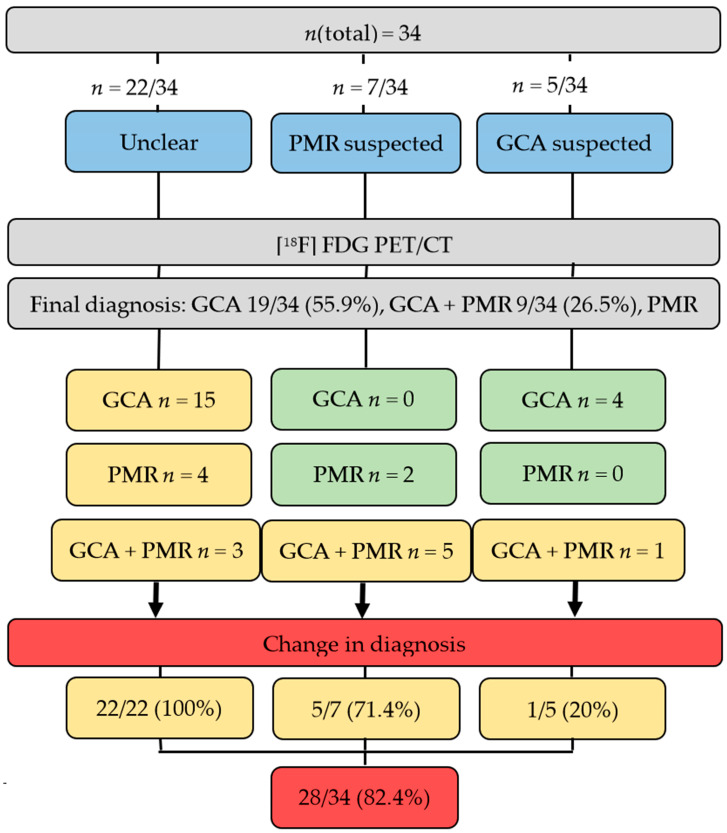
Thirty-four patients were examined by [^18^F]fluorodeoxyglucose (FDG) positron emission tomography/computed tomography (PET/CT) with unclear diagnosis or suspected giant cell arteritis (GCA) or polymyalgia rheumatica (PMR). In 28/34 (82.4%), [^18^F]FDG PET/CT was able to establish the diagnosis (red). In the remaining 6/34 (17.6%), [^18^F]FDG PET/CT had no additional benefit (green). The greatest benefit was recorded in an unclear situation (22/22 [100%]), with the vast majority having a final diagnosis of GCA (15/22 [68.2%]), followed by PMR in 4/22 (18.2%) and GCA + PMR in the remaining 3/22 (13.6%) (yellow).
